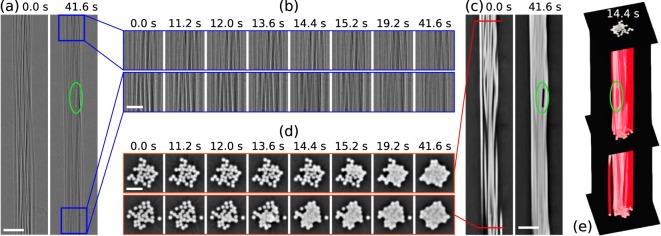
Time series of scans showing the water uptake dynamics of the yarn. (a) Radiographic projection images show the whole imaged yarn section at the beginning (0.0 seconds, dry) and the end (41.6 seconds, nearly completely wetted) of the uptake process. Scale bar: 200 *μ*m (b) Magnified sections of the radiographic images, indicated by the blue boxes in (a), at several time points during the scan at the top (upper line) and bottom (lower line) of the sample. Scale bar: 100 *μ*m (c) Vertical centre slice through the phase contrast reconstruction of the yarn sample at the beginning and the end of the scan series. Scale bar: 200 *μ*m (d) Horizontal cuts, indicated by the red line in (c), through the reconstructed volume at the top and bottom at the same time points shown in (b). Scale bar: 100 *μ*m (e) Rendering of the reconstructed volume at an intermediate time point during the uptake process, showing one vertical and three axial slices as well as a semi-transparent red isosurface outlining the volume of the combined yarn and water volume. Green outlines: Air bubble remaining in the yarn structure even at the end of the scan series.
